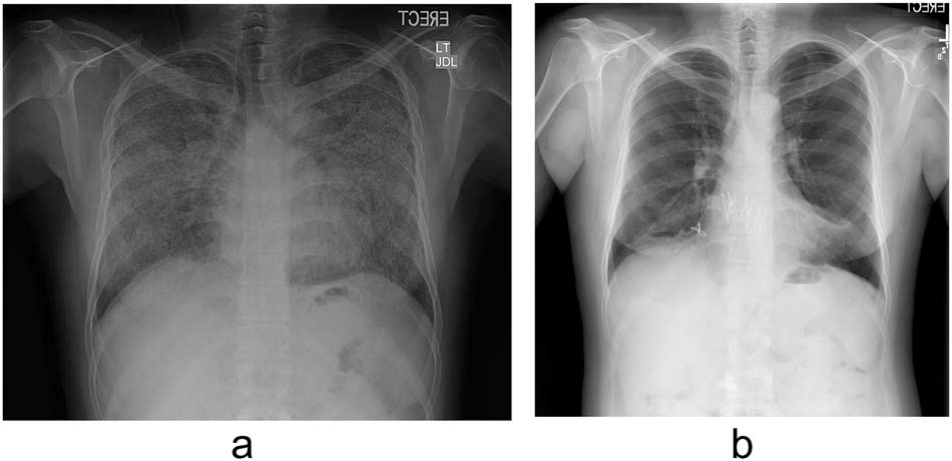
(a) Chest radiograph shows extensive calcification all over both lungs’ fields. (b) Chest radiograph after the transplant.
